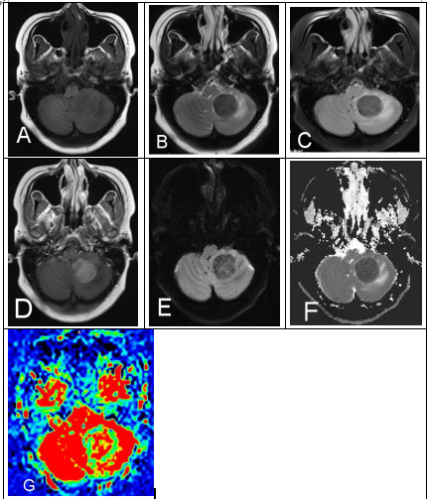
A 29-year-old female with a history of AML in remission presents with headaches, nausea and vomiting. (A) MRI demonstrated left cerebellum mass unenhanced T1WI, (B) T2WI, (C) FLAIR isointense-to-mild hypointense and (D) CE-T1WI showed homogenous hyperintensity. (E) DWI and (F) ADC map demonstrated mild-to-moderate restricted diffusion. (G) ASL showed mild hypointensity with mild surrounding vasogenic edema and mass effect.
